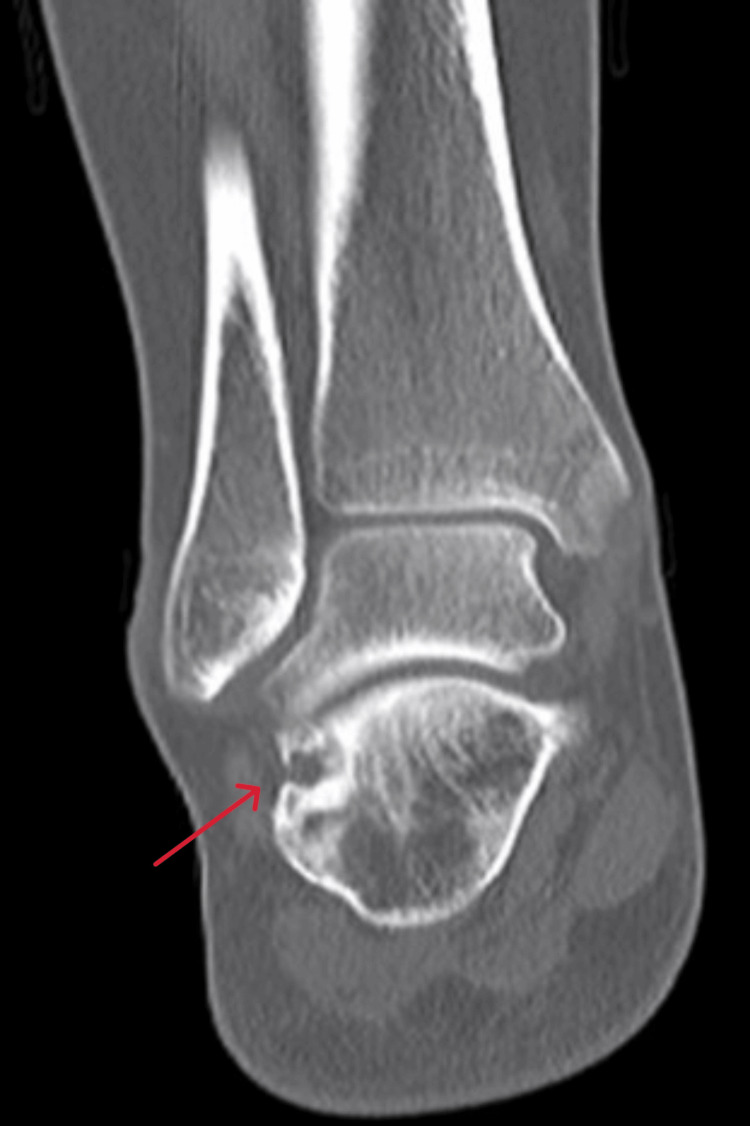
Coronal view CT scan of the right ankle at one-and-a-half-year follow-up CT: computed tomography
